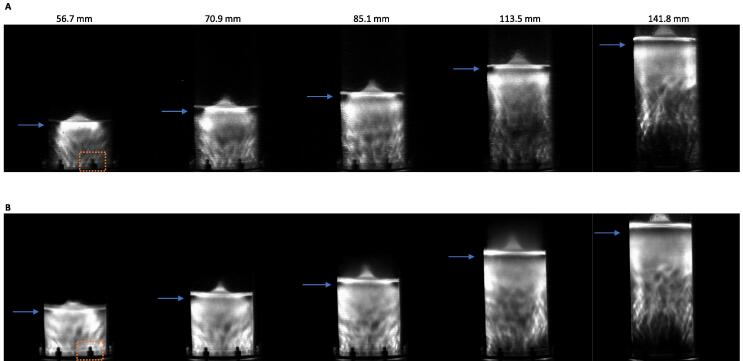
Representative sonoluminescence (A) and sonochemiluminescence (B) images (one of three repeats) for sonolysis of Milli-Q water using 410 kHz ultrasound at 200 W/L applied power, in liquid heights of 56.7, 70.9, 85.1, 113.5 and 141.8 mm, respectively. Note the bold shadows seen at the bottom of the photographs are due to screws and nuts which affix the transducer to the reactor base (examples shown with orange dotted box).
